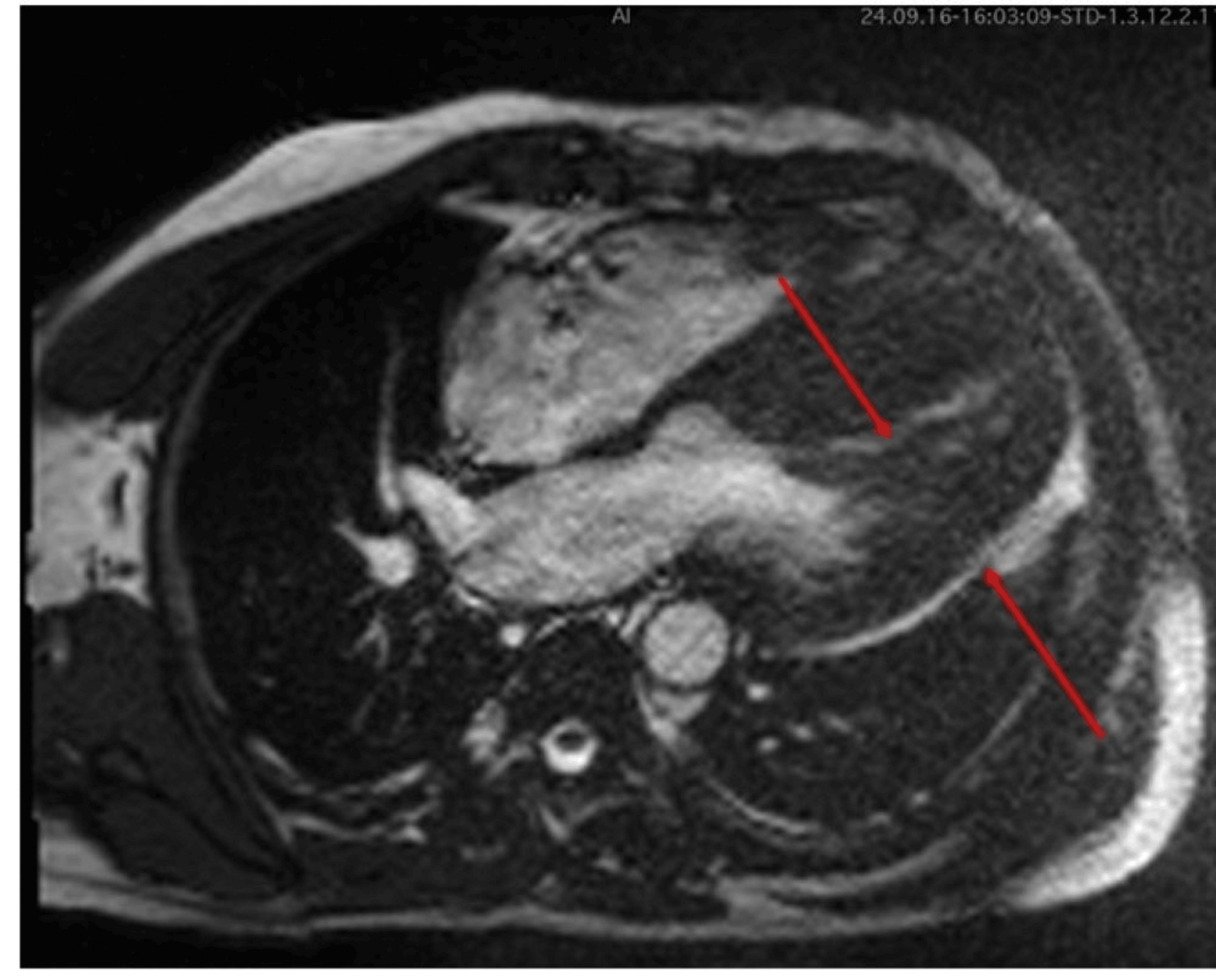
Cardiac MRI four-chamber view showing septal thickening and a small LV cavity (red arrows). LV: left ventricular.
